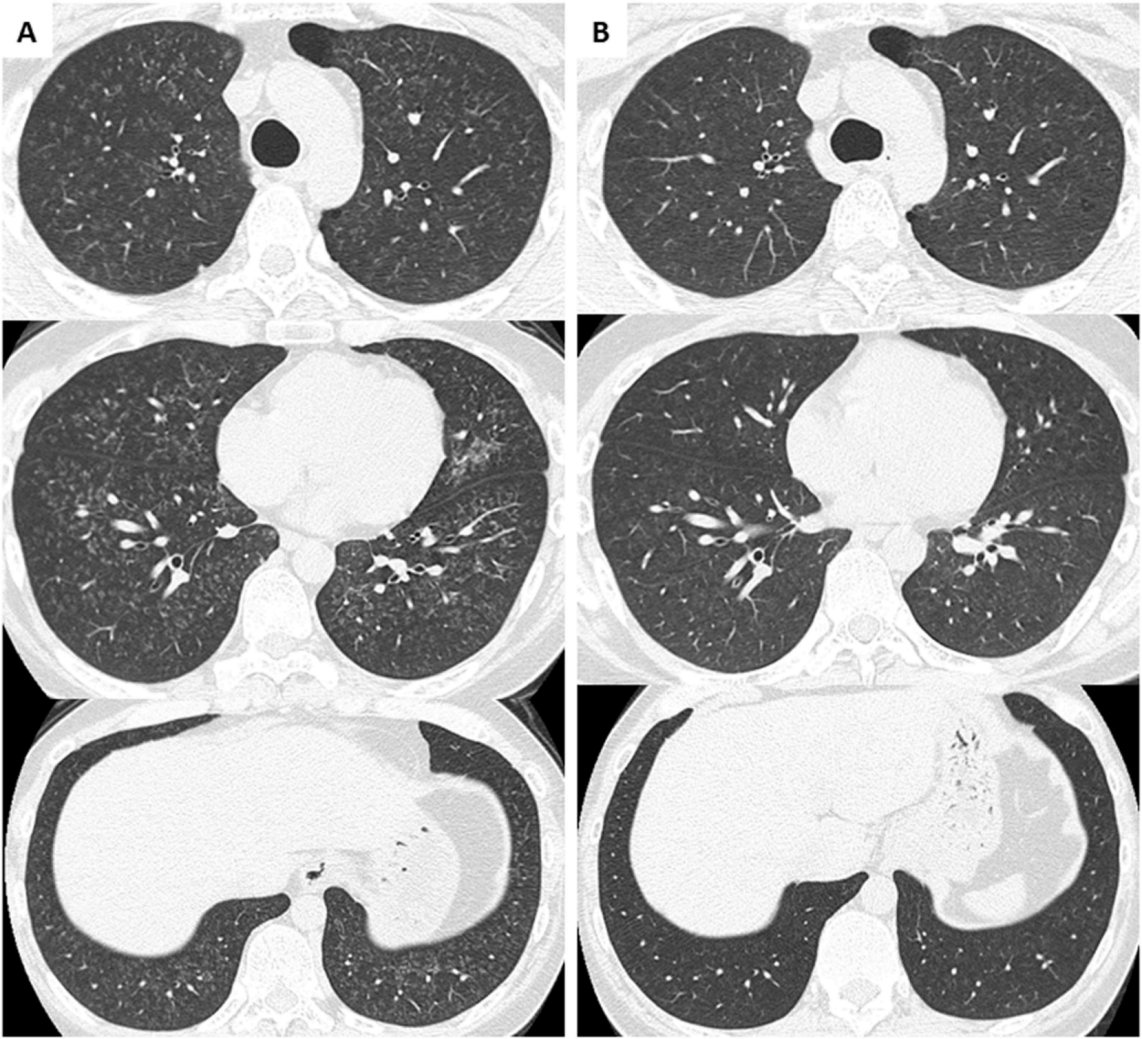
Chest computed tomography showing improvement on day 44 (A) and further improvement on day 460 (B).
